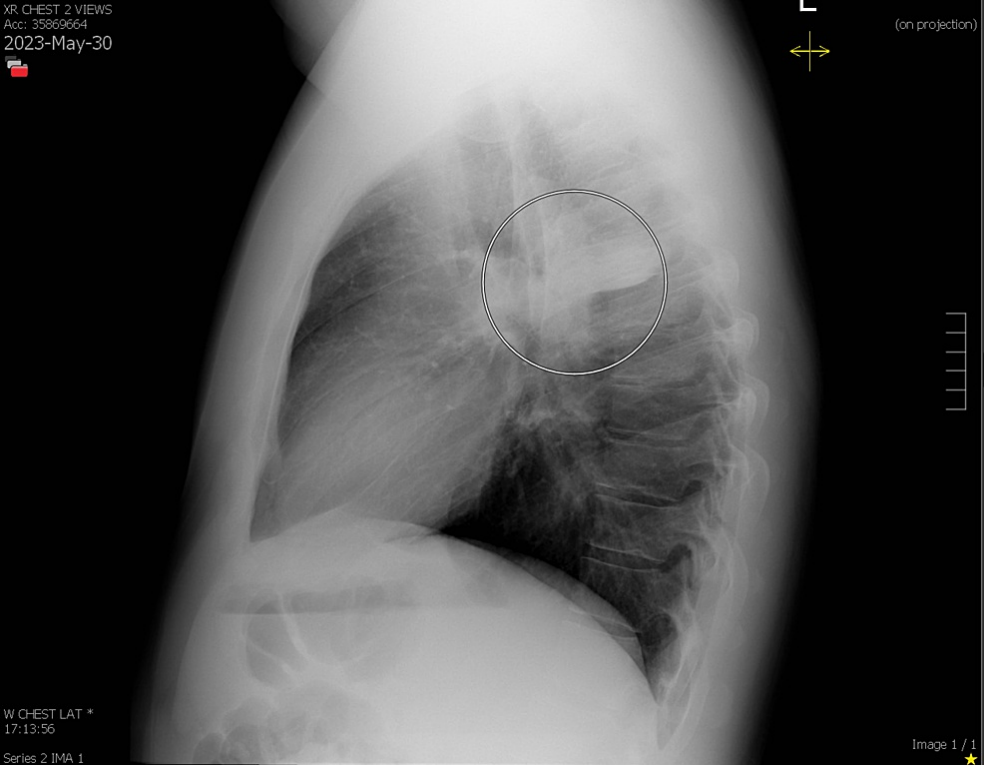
Chest X-ray depicting right upper lobe consolidation (lateral view)
